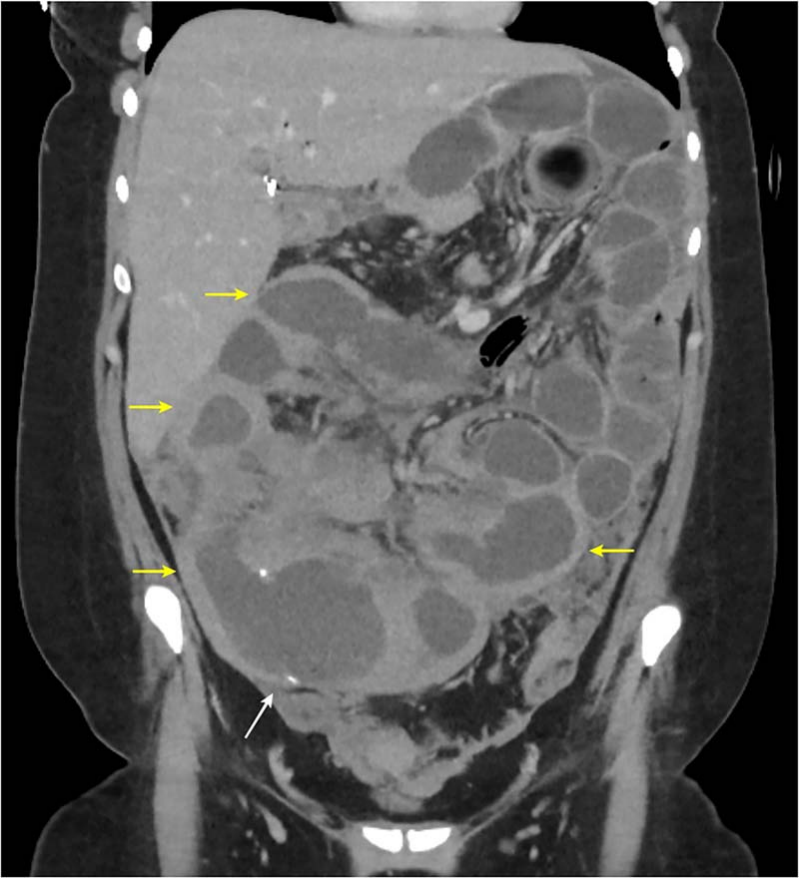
Abdominal CT coronal slice demonstrating multiple loops of dilated fluid filled small bowel with significant wall thickening.
